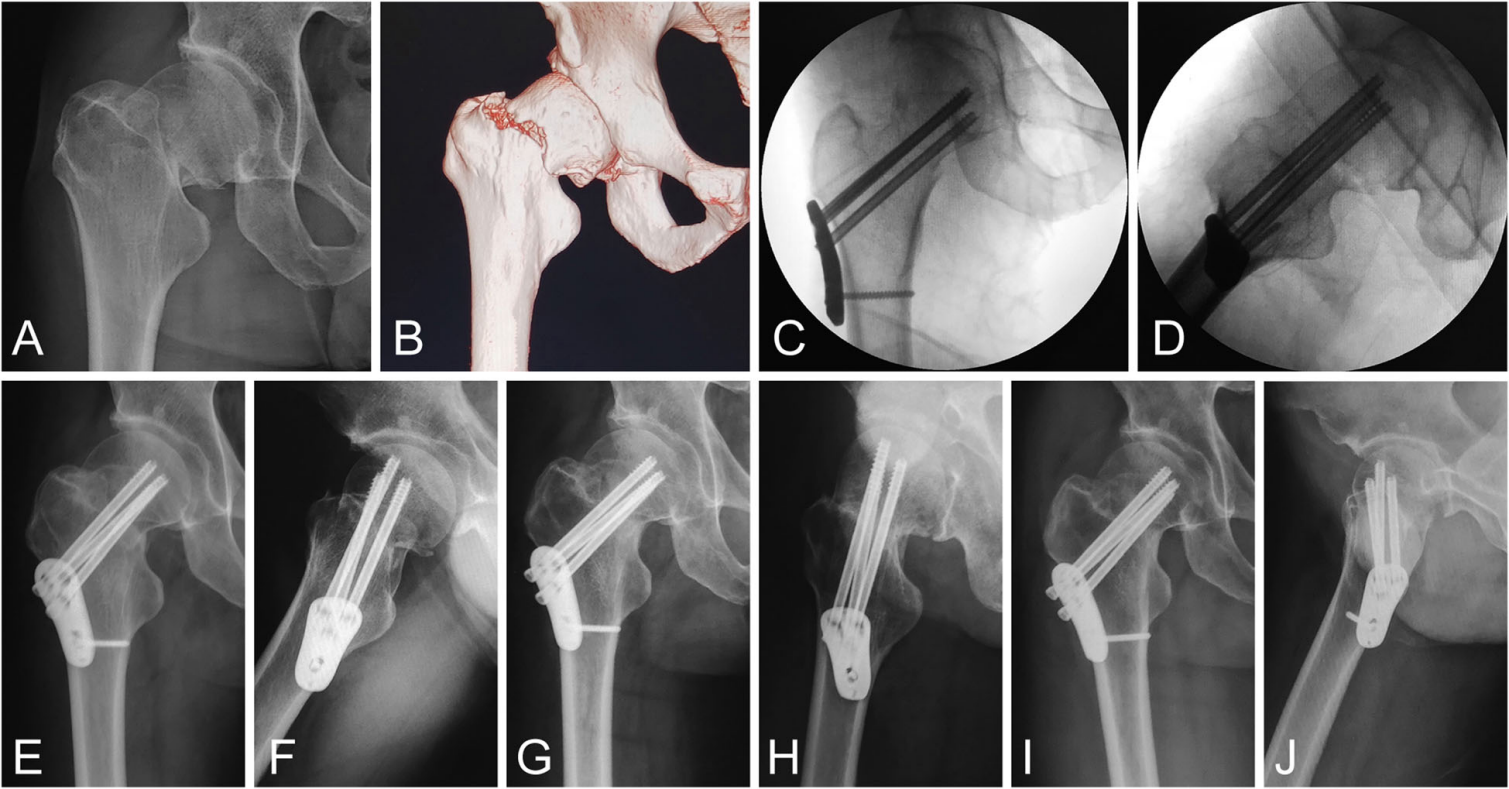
Proximal femoral plating of a 41-year-old female with retroverted femoral neck fracture (Garden type III). a Preoperative X-ray. b, c Preoperative CT scans and three-dimensional reconstruction. Postoperative radiographs at 1 day (d, e) (arrowhead: anterior long-threaded screw), 4 months (f, g), 6 months (h, i) (arrowhead: anterior long-threaded screw), and 12 months (j, k) (posterior tilt: − 3.3°)
